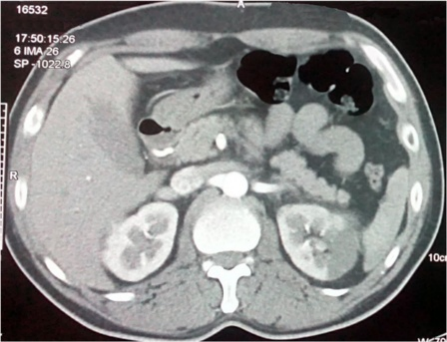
Axial contrast-enhanced computed tomography of abdomen and pelvis showing a non-enhancing, hypodense, sharply demarcated, wedge-shaped area involving the cortex of the patient’s left kidney suggestive of left renal infarction
